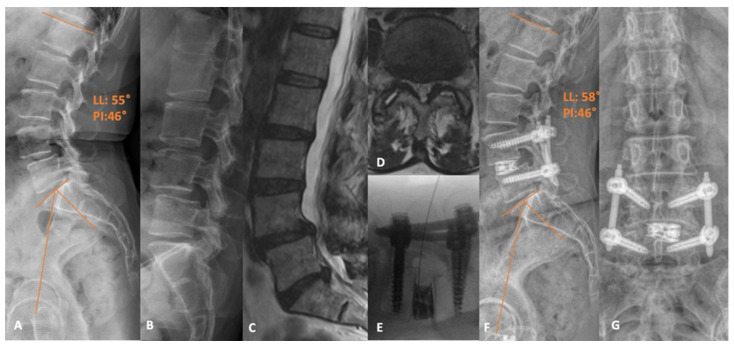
(A) Preoperative standing X-ray demonstrating the L4-5 grade 1 spondylolisthesis, with (B) flexion films demonstrating dynamic instability. (C) Sagittal and (D) axial T2-weighted MRI slices revealing the advanced central and bilateral recess stenosis at the L4-5 level. (E) Intraoperative fluoroscopic image following cage placement with a guidewire within the disc space. (F) Postoperative lateral and (G) AP X-rays supporting a satisfactory appearance of the construct. LL: Lumbar lordosis. PI: Pelvic incidence.
